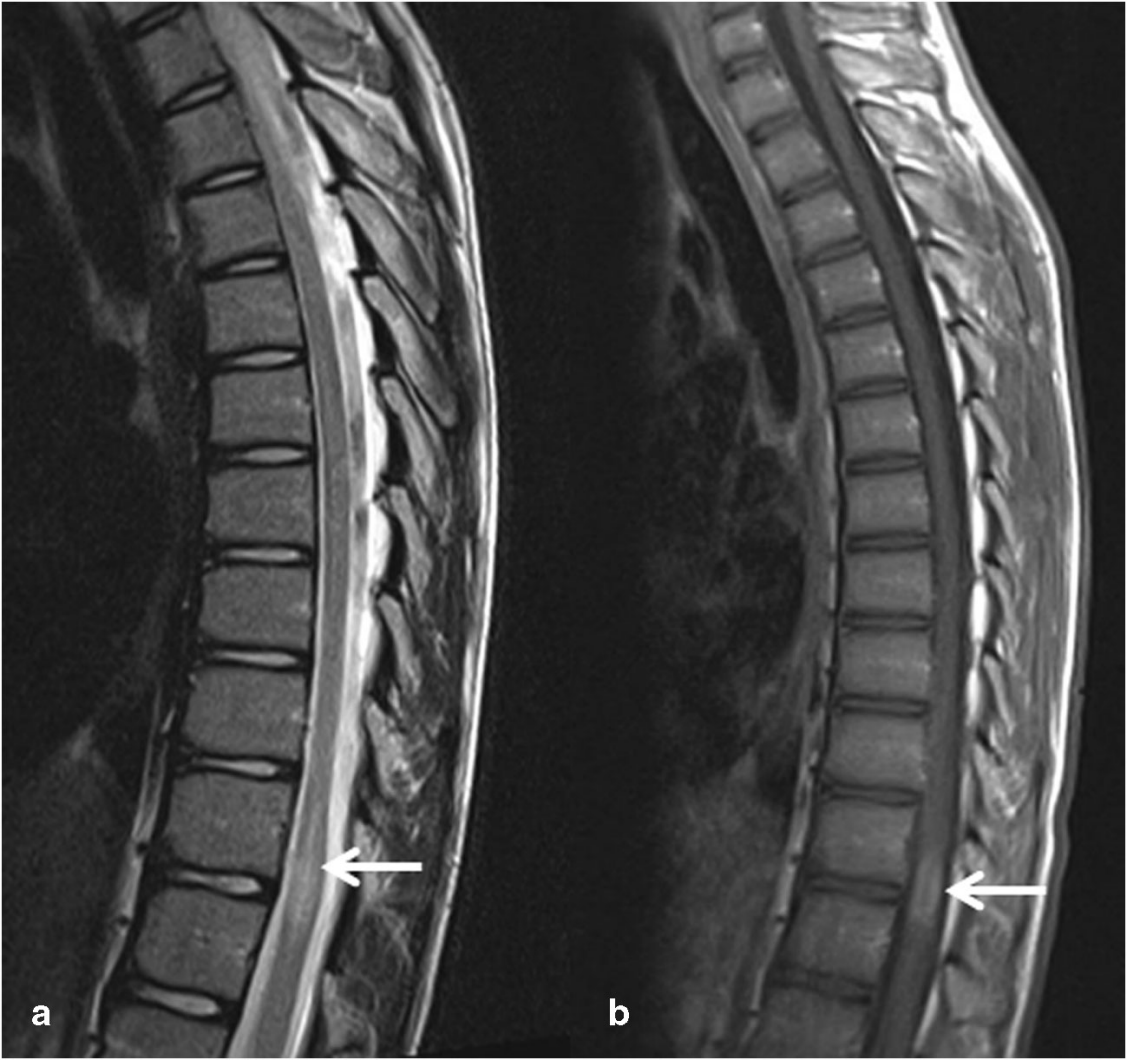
MRI of the spinal cord in a 15-year-old boy with acute transverse myelitis, eventually diagnosed with relapsing remitting multiple sclerosis. a Sagittal T2 showing focal swelling of the spinal cord at level Th11–12. b Sagittal T1 showing contrast enhancement of the lesion
